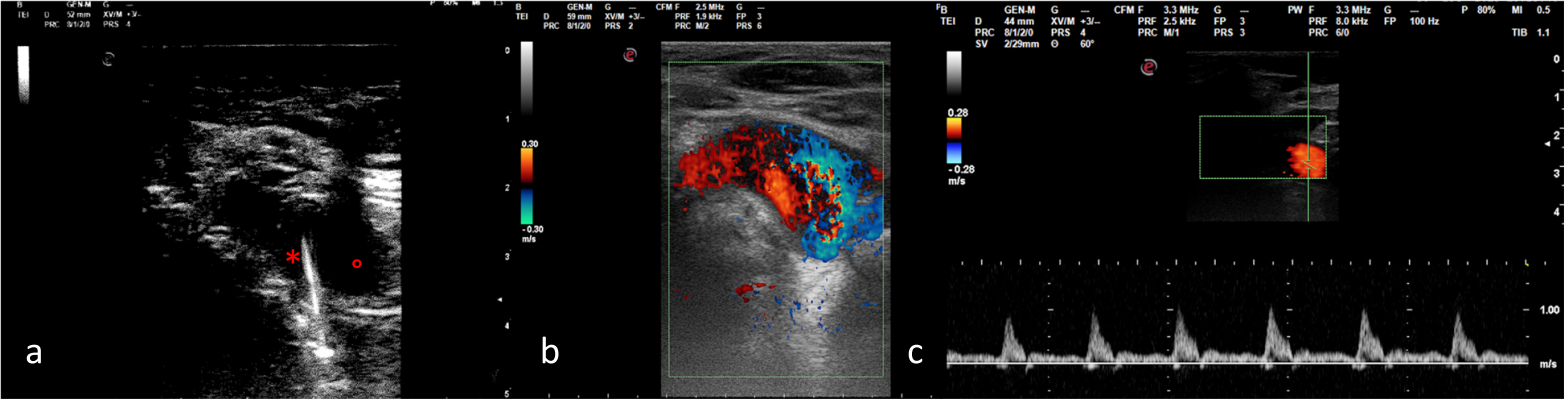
panel a, suprasternal notch acoustic window, short axis ultrasound image showing the innominate artery crossed by the guide wire. * guidewire, ° innominate artery; panel b, innominate artery color Doppler appearance; panel c, innominate artery pulsed-wave Doppler
